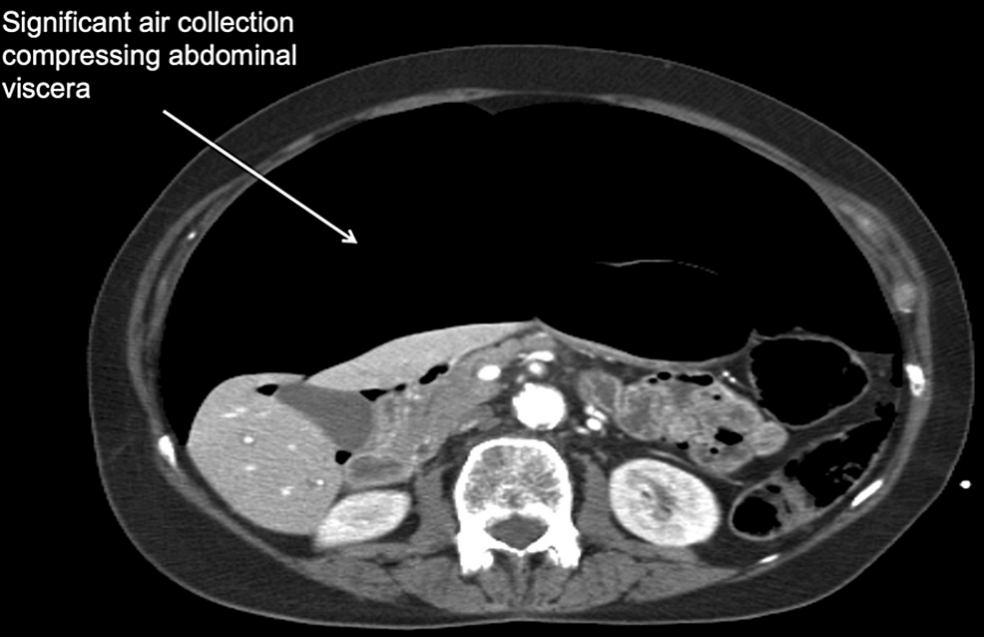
Axial CT scan image showing a significant collection of air compressing the abdominal viscera.
